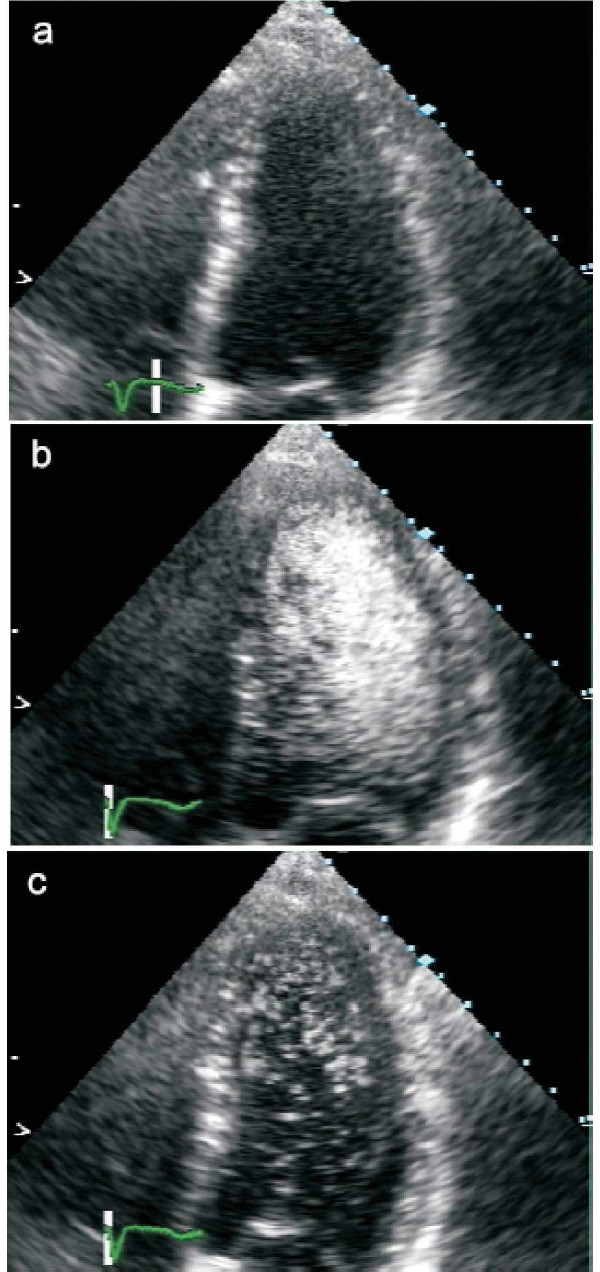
Apical 4 chamber view with LK565 Apical 4 chamber view before (a), 1 minute (b), and 30 minutes (c) after intravenous LK565 application (4 mg/kg BW) and intermittent ultrasound exposure. Device: SONOS 5500, S3 Probe, harmonic
